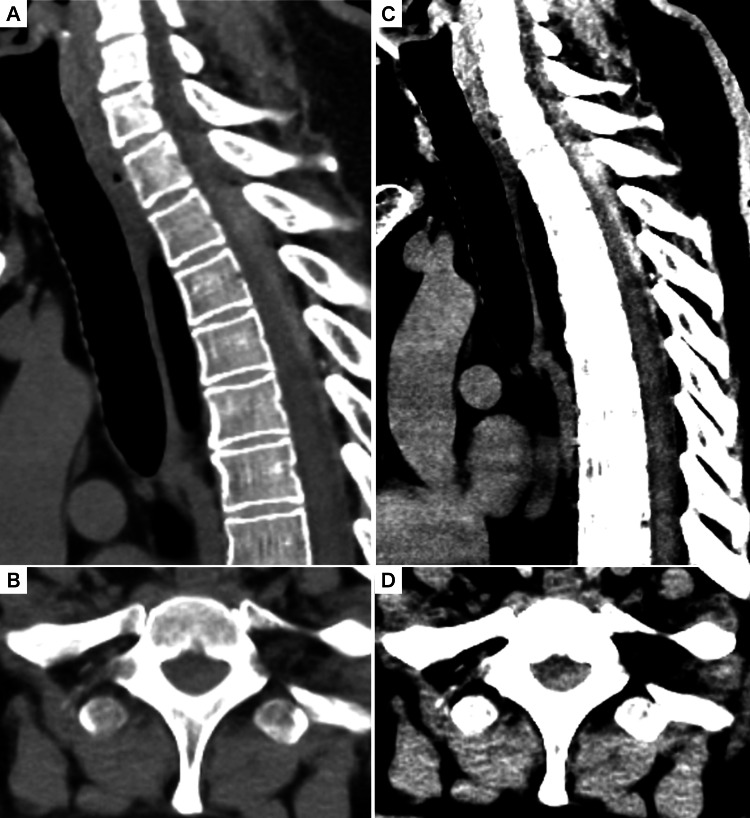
Plain trunk CT (reconstructed from 1-mm source data) (A, B) Sagittal (A) and axial (B) reconstructions of a non-contrast trunk CT on a standard mediastinal window (WL 40, WW 320). The subdural hematoma is poorly defined and nearly isodense with the spinal cord. (C, D) The same sagittal (C) and axial (D) views reviewed with high-contrast brain window settings (WL 35, WW 80).
